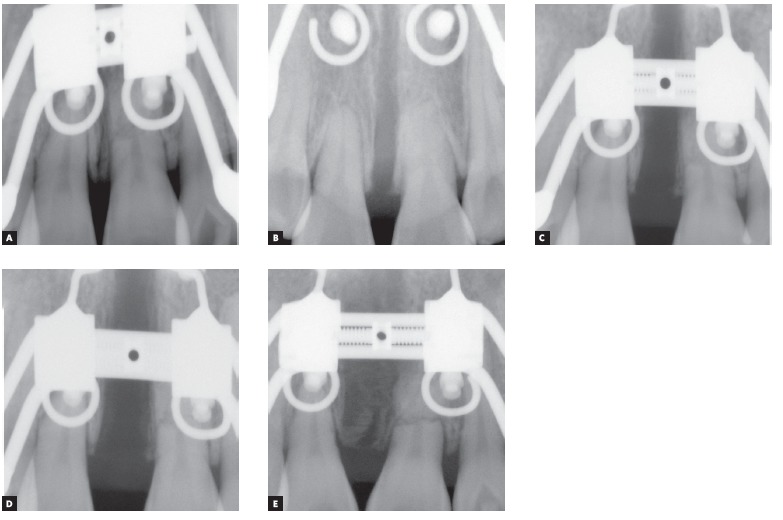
Upper incisors periapical radiographs: A) before expansion, B-D) during active expansion and E) after activation period.
